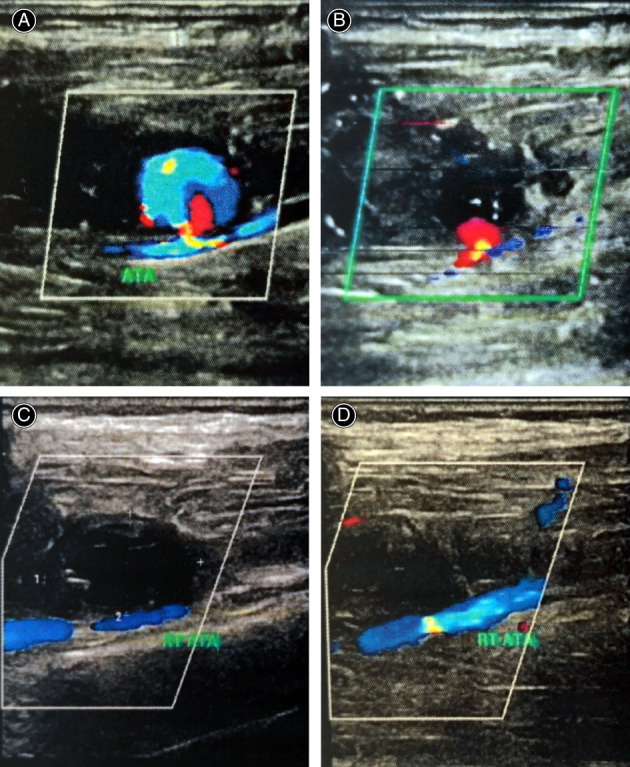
A 43‐year‐old patient with a mass on the lower leg. (A) Color Duplex sonography demonstrates an anterior tibial PA. (B) Color Duplex sonography shows thromboembolization in the sac during UGTI. (C, D) Color Duplex sonography shows the thrombosed PA with no blood circulation signal in the sac after operation
